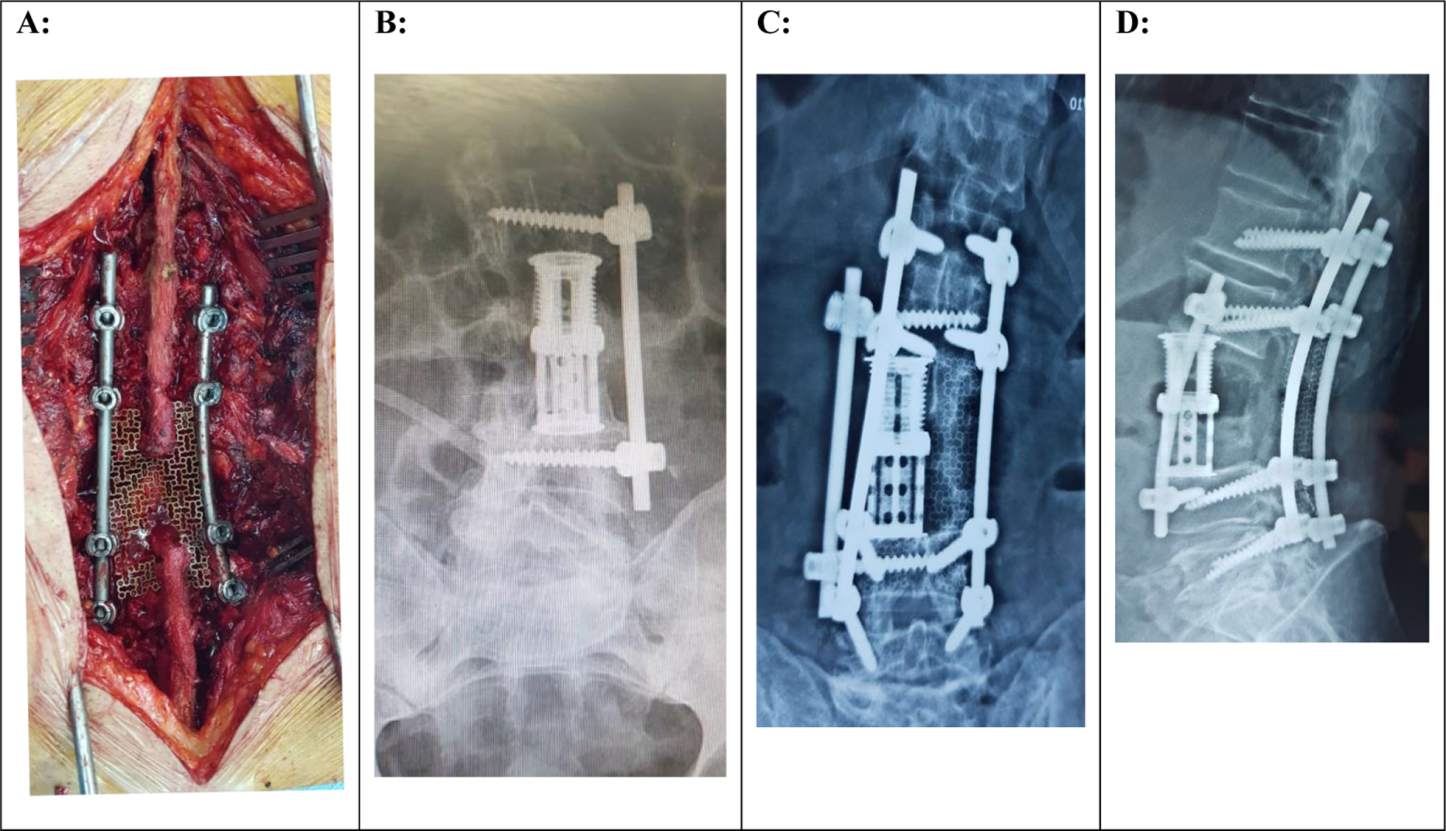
Intraoperative photograph (A) showing titanium mesh placed on the post-laminectomy defect. X-ray imaging after first surgery (B), anteroposterior and lateral lumbar spine radiographs (C and D) at the last follow-up
